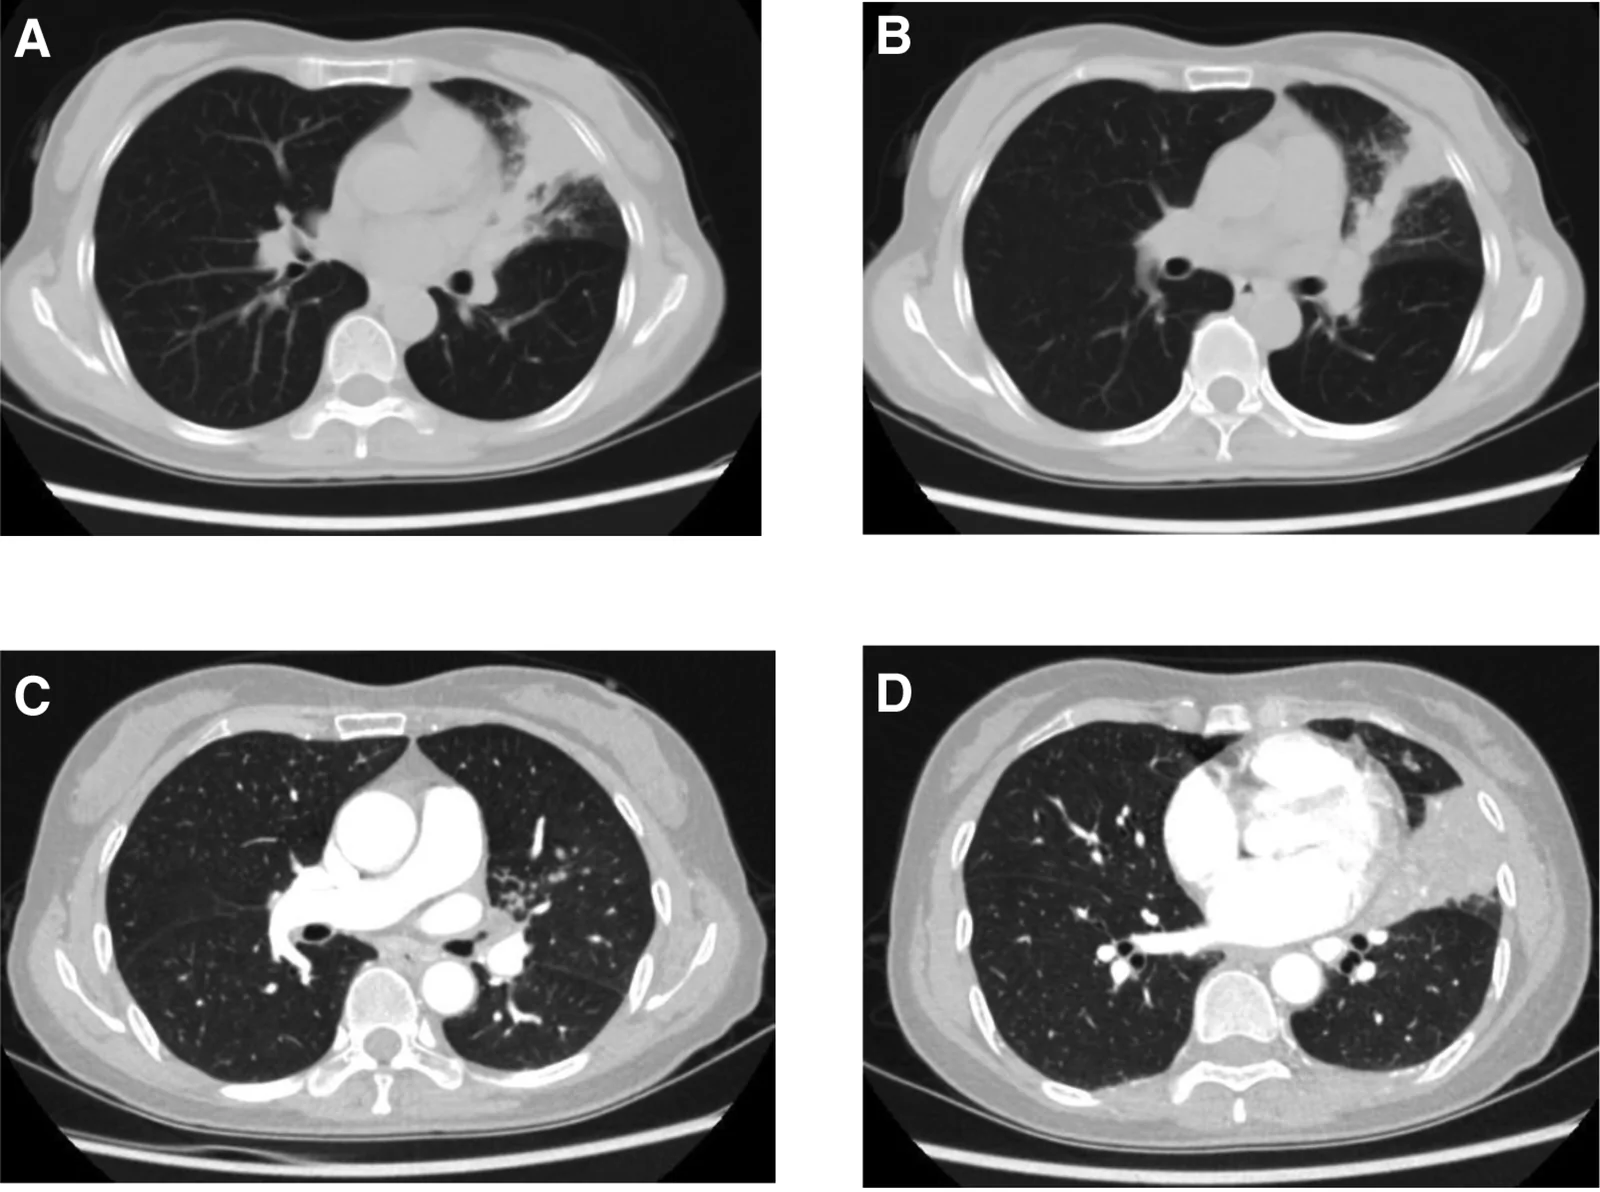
(A) and (B) Chest CT five years ago. (C) and (D) The Chest CT at admission.
